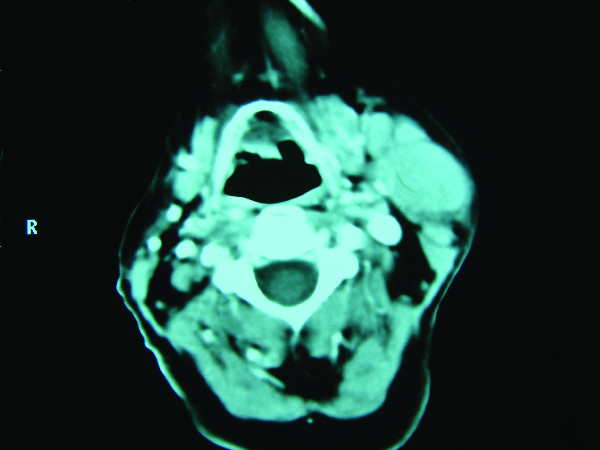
Preoperative CT scan of the tumor of the left parotid gland.
